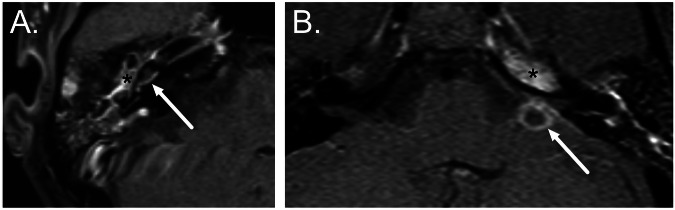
Medial extension of AOM on axial post-contrast T1-weighted MRI. A Acute labyrinthitis: AOM with inflammatory enhancement in the middle ear cleft (asterisk) associated with abnormal enhancement in the cochlea (arrow) indicating labyrinthitis. B Petrous apicitis with Gradenigo syndrome: AOM with inflammatory enhancement extending medially into the petrous apex (asterisk) with the development of an intracranial abscess adjacent to the petrous ridge (arrow)
